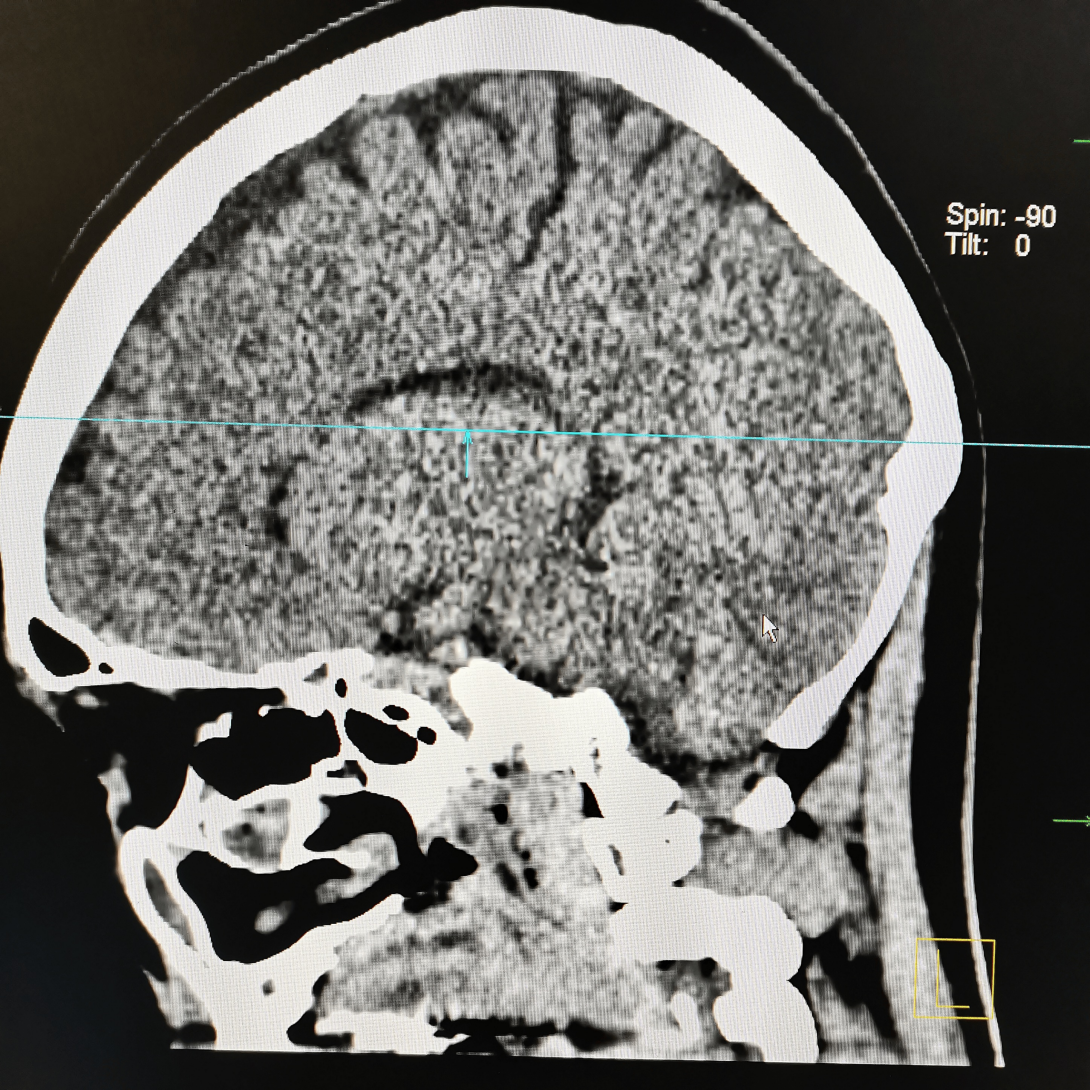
CT brain (sagittal view) shows no significant abnormalities
